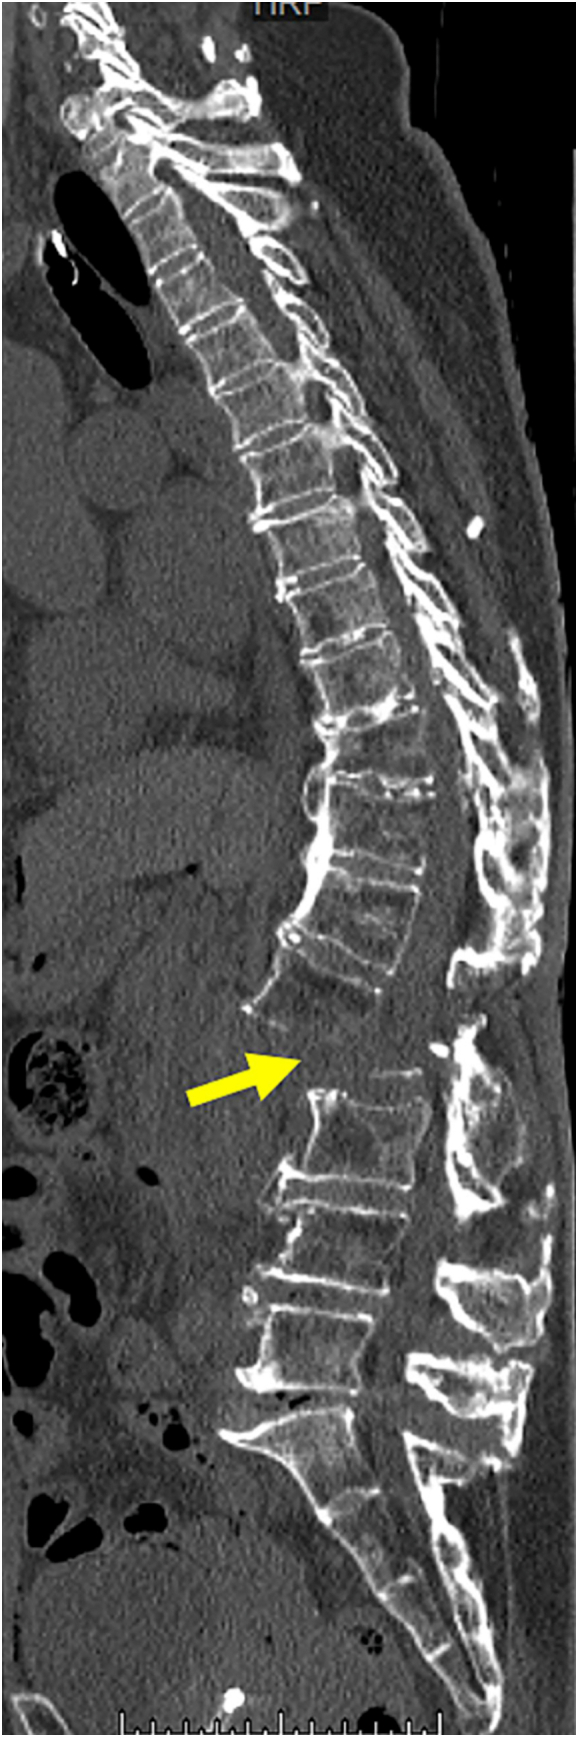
Contrast-enhanced computed tomography showing a complete displaced fracture of the second lumbar vertebra (arrow).
